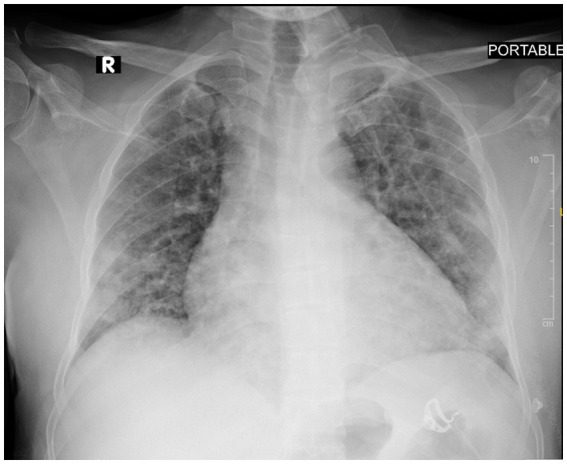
Portable anteroposterior chest radiograph showing diffuse bilateral peripheral patchy ground-glass opacities consistent with viral pneumonitis.
